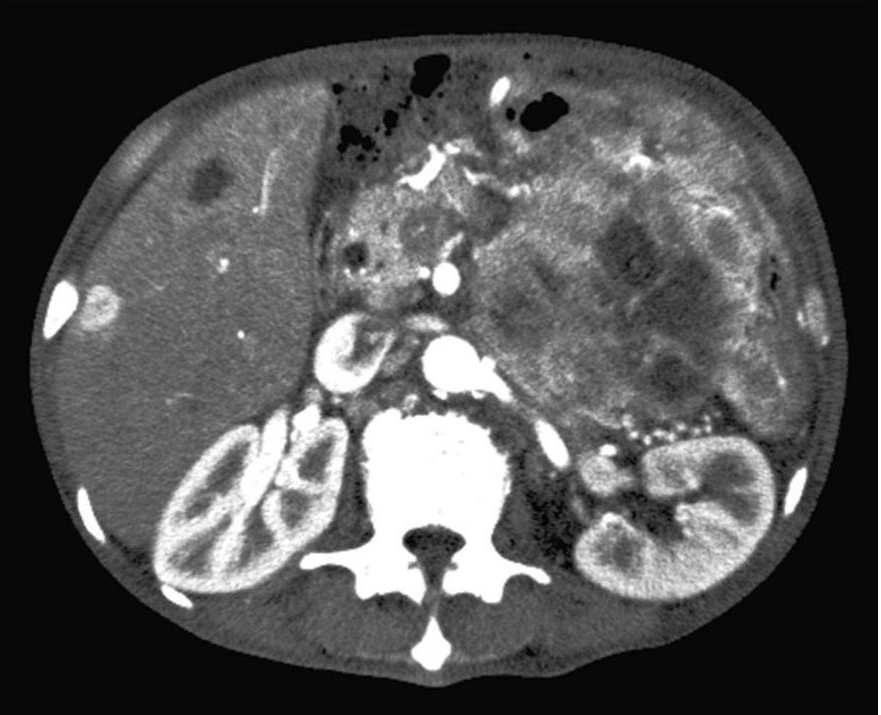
Computed tomography showed an unevenly enhanced mass 10 cm in diameter in the patient's left upper abdomen.
